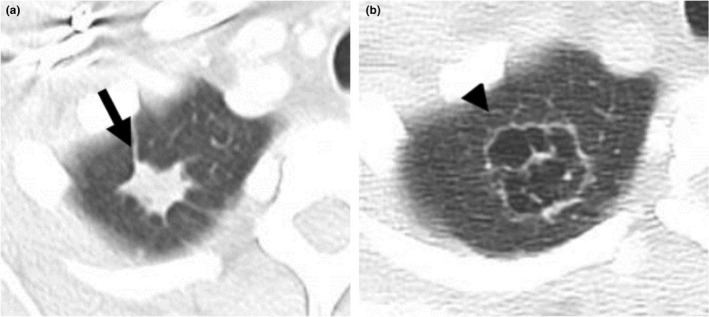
A 34‐year‐old female with a history of GPA was found to have an irregular nodule (black arrow) in the right lung apex on CT (a). This lung nodule showed cavitation (arrowhead) on the follow up CT 3 months later (b), which is in keeping with the evolution of GPA.
